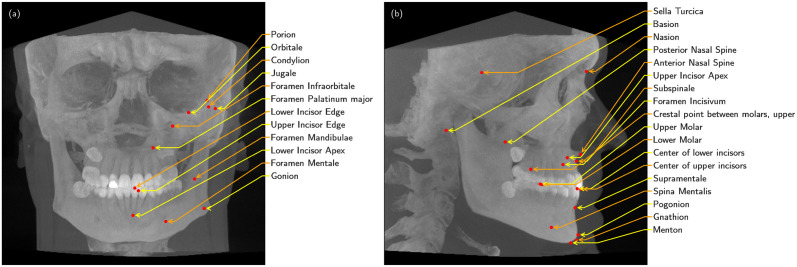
Visualization of the annotated landmarks overlaid on a maximum intensity projection of a CBCT. (a) Left side landmarks from the laterally symmetric pairs. (b) Midline landmarks.
